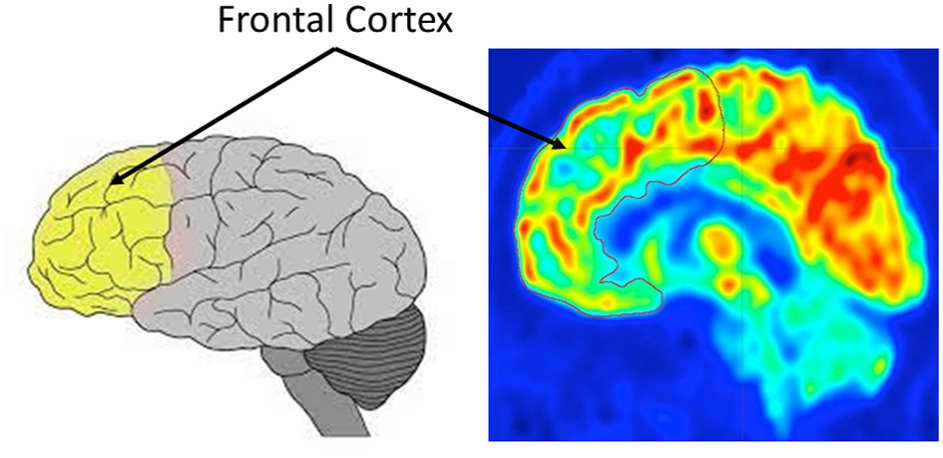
The frontal cortex in the brain: the left is anatomical location, and the right is for ^18^F-FDG positron emission tomography (PET) imaging.
